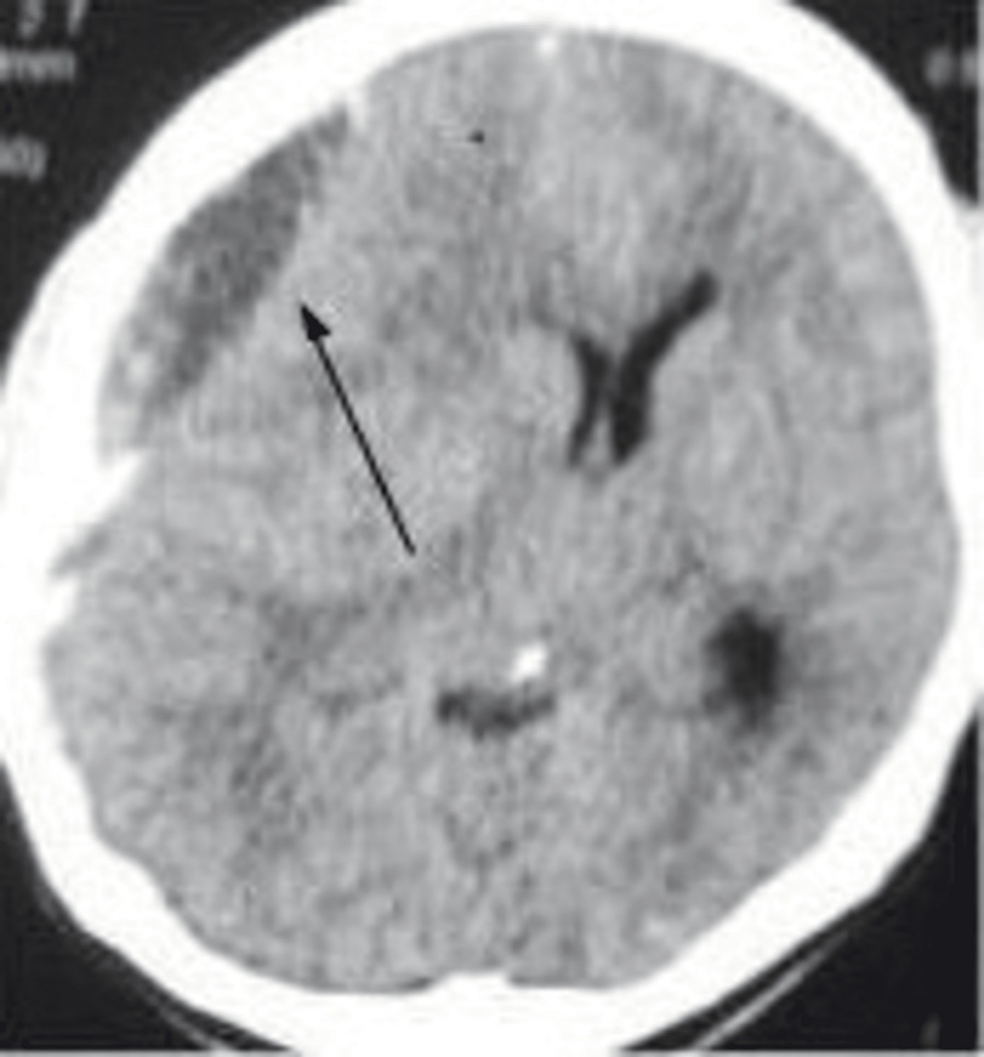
A CT scan of the brain with axial view showing a mixed density picture of the hyperdense and hypodensce crescent-shaped lesion in the right fronto-parietal region with a midline shift (arrow)
